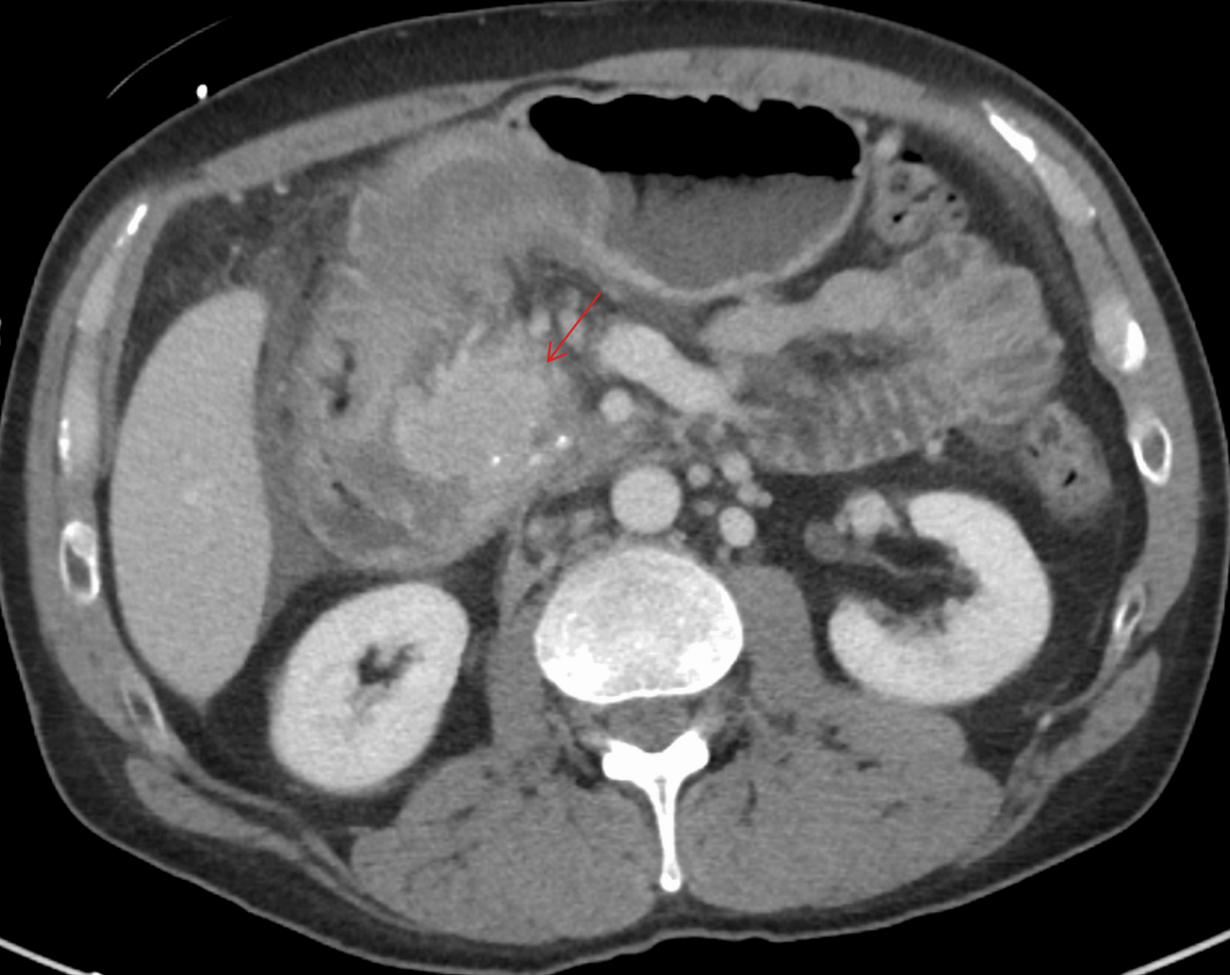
CT abdomen with IV contrast (axial view) demonstrating progression of pancreatitis with an indeterminate mass in the pancreatic head (red arrow) and associated duodenal inflammation.
